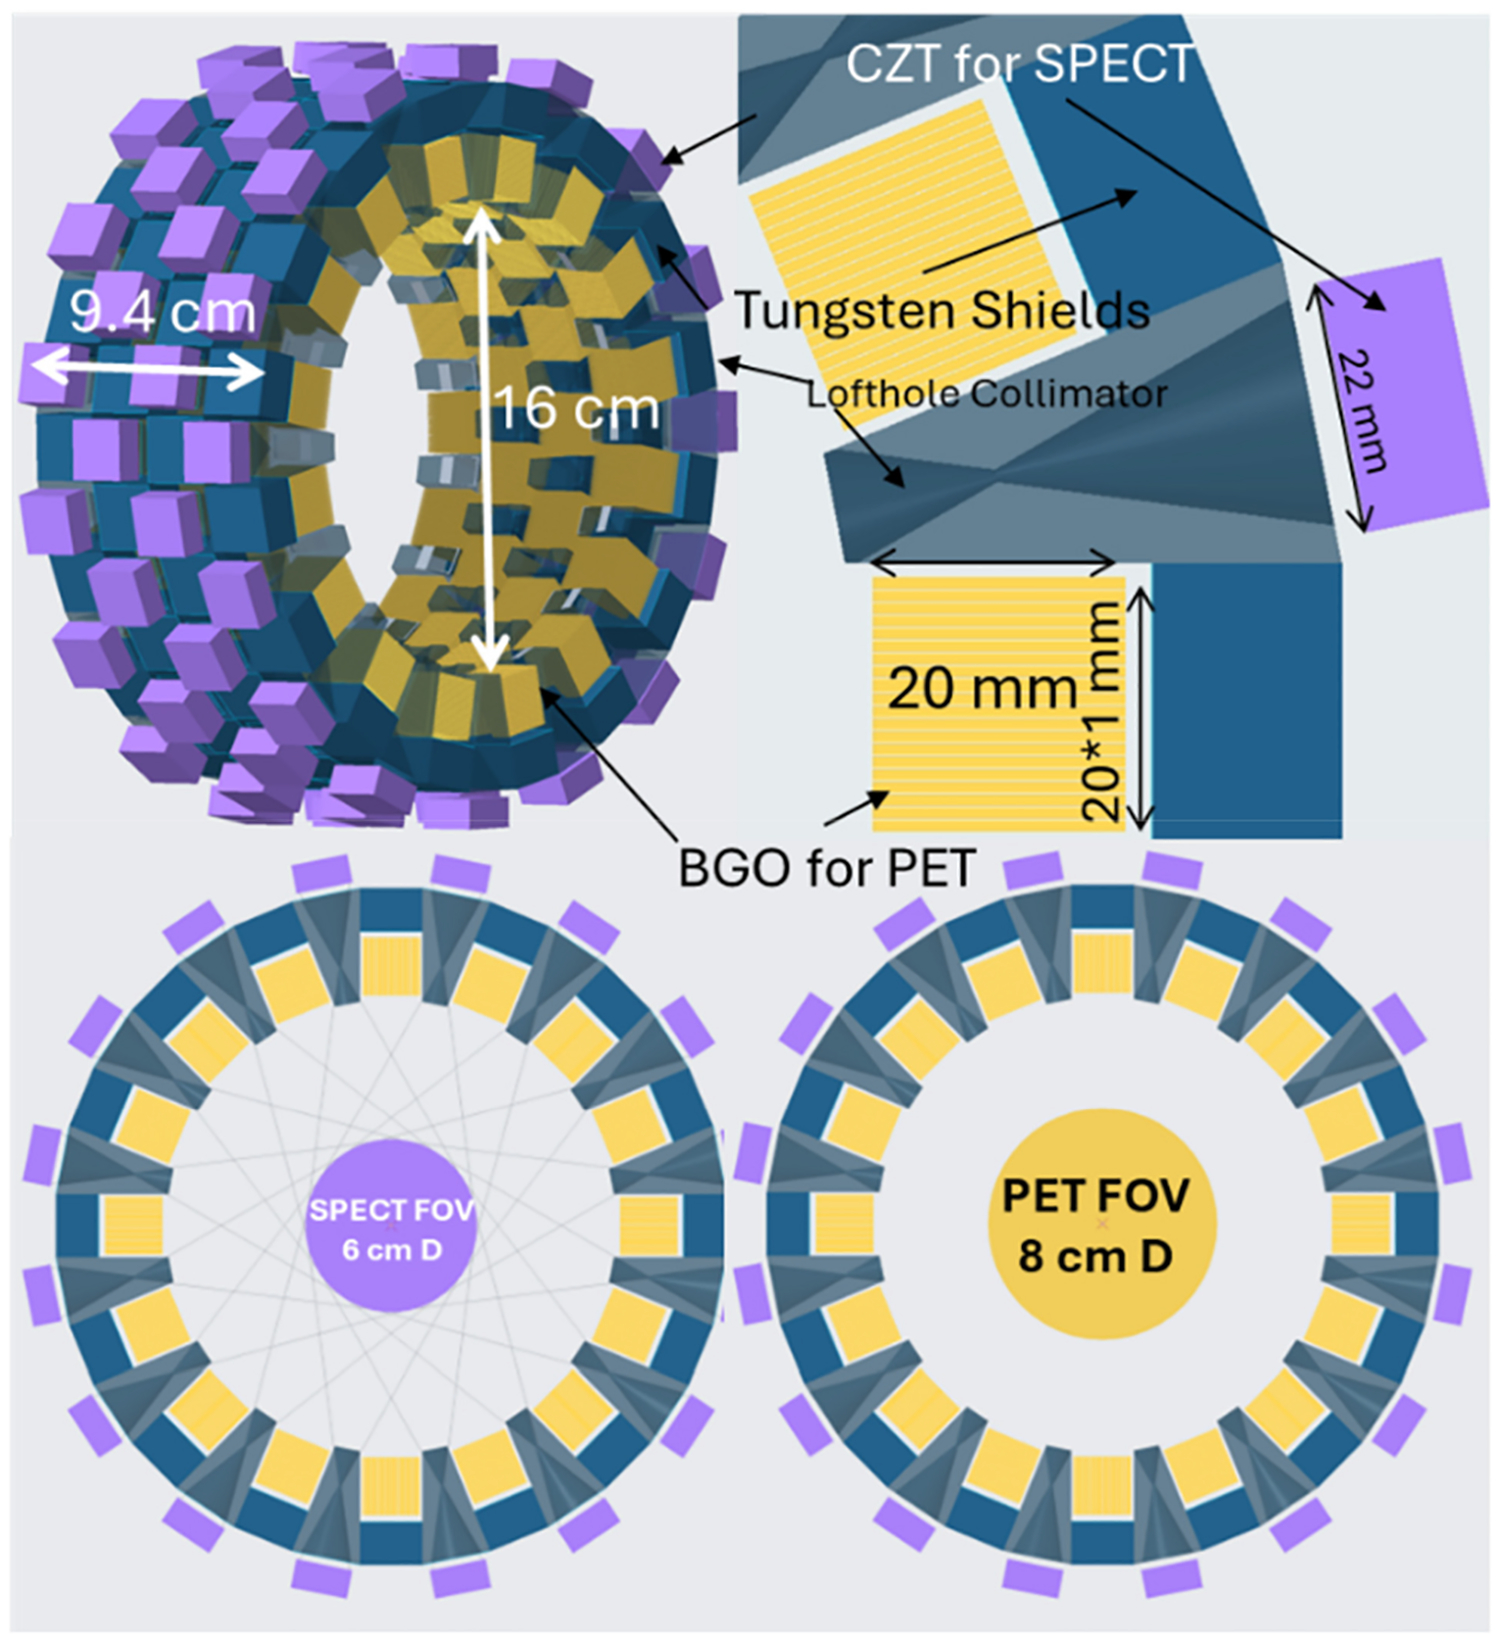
Detailed design of the proposed preclinical PET/SPECT system. A zoomed-in view shows the dimensions of PET modules (20 × 20 × 20 mm3) and SPECT modules (22 × 22 × 10 mm^3^) with tungsten lofthole collimators and shielding. The transverse FOV for SPECT is 6 cm in diameter, and for PET is 8 cm in diameter.
